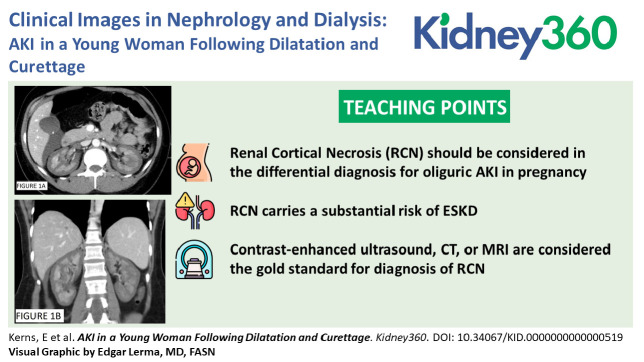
A 31-year-old woman with no medical history presented with hemorrhagic shock after dilation and curettage at 17 weeks gestation due to intrauterine fetal demise. Laboratory test results were consistent with disseminated intravascular coagulation. Serum creatinine was 2.0 mg/dl from a previous baseline of 0.6 mg/dl. She was transferred to the medical intensive care unit and treated with blood transfusions, fresh frozen plasma, and cryoprecipitate along with oxytocin, misoprostol, and thromboxane. She underwent uterine artery embolization because of a large intrauterine hematoma. During the next 6 days, serum creatinine increased to 13 mg/dl accompanied by anuria. A contrast-enhanced computed tomography (CT) scan of the abdomen and pelvis revealed bilateral reverse nephrostograms (reverse rim signs), with the renal cortex appearing hypodense to the renal medullae. There was a nonobstructing inferior vena cava thrombus and a right gonadal vein thrombus. Renal cortical necrosis (RCN) was diagnosed. She started hemodialysis for uremic symptoms and remained dialysis dependent for approximately 2 months. Six months after the diagnosis, the serum creatinine was 2.4 mg/dl with a creatinine clearance on 24-hour urine collection of 33 ml/min and subnephrotic proteinuria, 300 mg/d. The patient remains on low-dose loop diuretics and anticoagulation.
